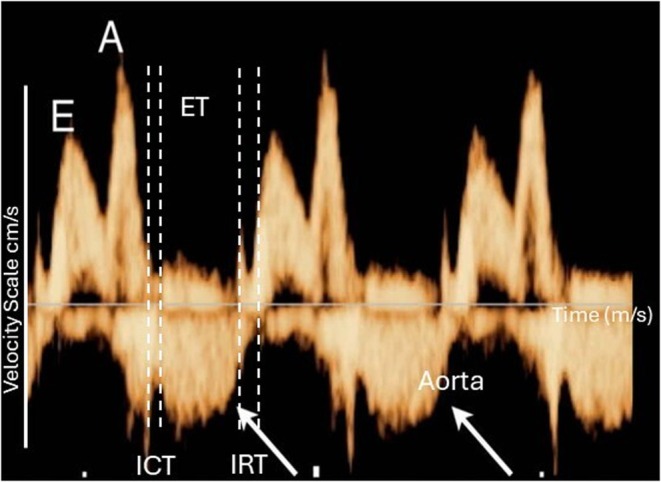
(A) Representative spectral Doppler images of left ventricular inflow and outflow obtained from the hearts of baboon offspring. The inflow Doppler demonstrates the early diastolic (E) and late diastolic (A) velocities across the mitral valve. Arrows indicate the aortic valve Doppler waveform. The interval between mitral valve closure and aortic valve opening represents the isovolumetric contraction time (ICT), the interval between aortic valve opening and closure represents the ejection time (ET), and the interval between aortic valve closure and mitral valve opening represents the isovolumetric relaxation time (IRT).
